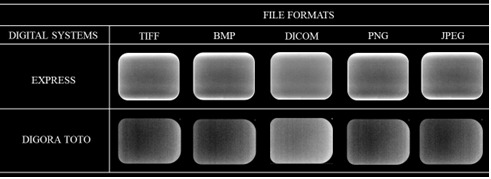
Radiographic images were acquired in the different digital radiographic systems with each image file format investigated.
